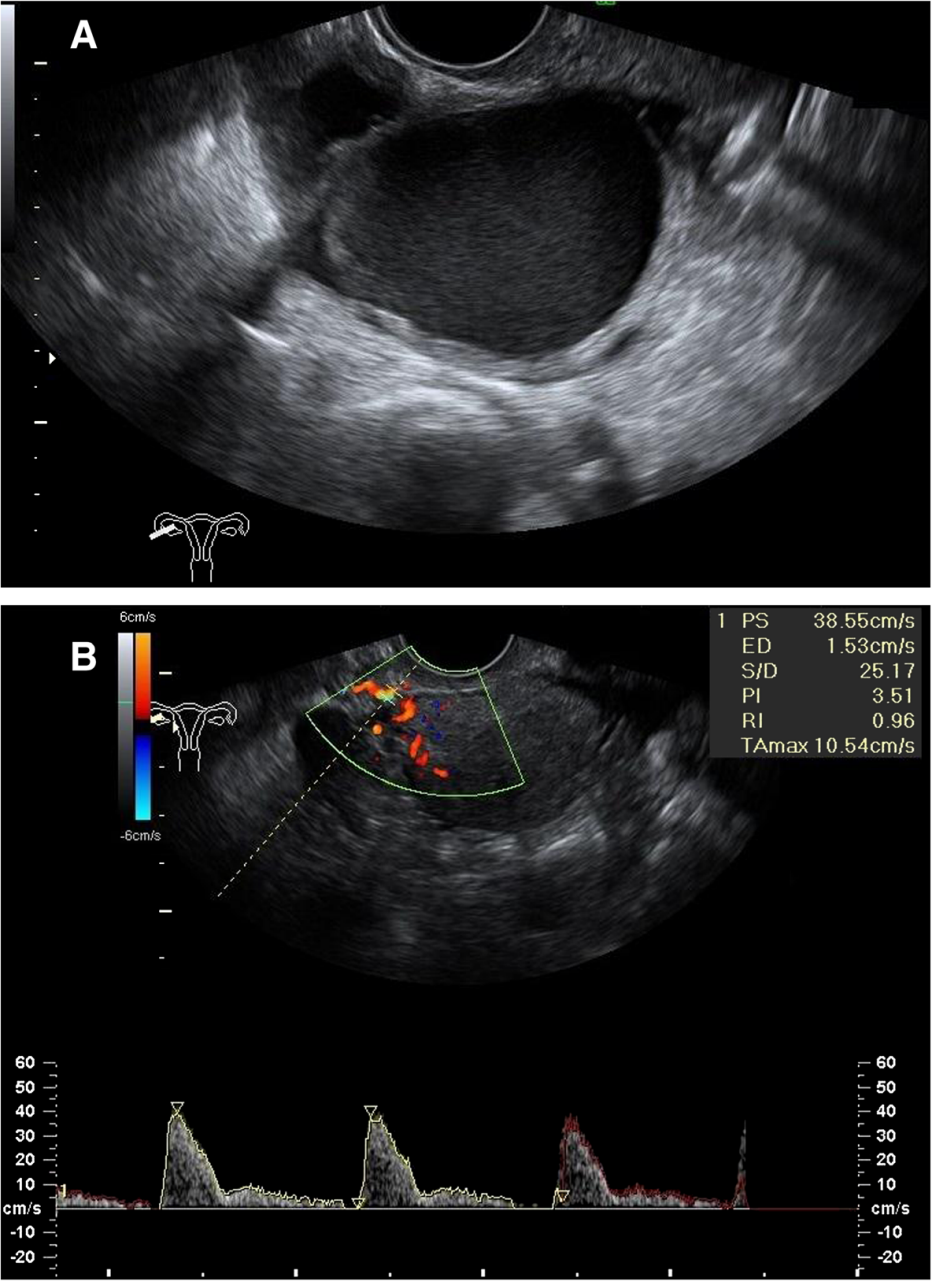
Transvaginal ultrasonography and color Doppler. (A) Right ovarian endometrioma and (B) Homolateral pre-operative Doppler flow alteration (RI = 0.96).
